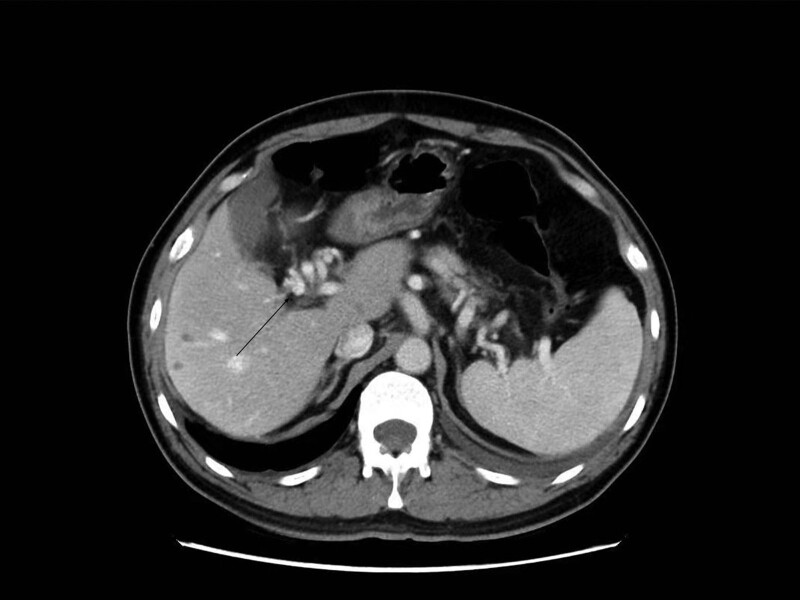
Axial CT scans showed numerous collateral vessels in the liver hilum (arrow).
